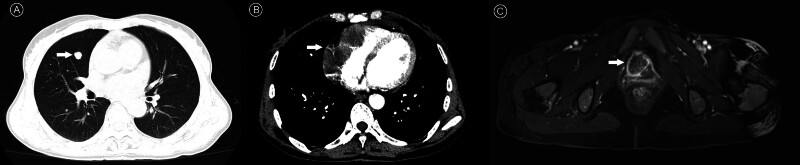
Baseline imaging evaluation of pulmonary, cardiac, and urethral involvement prior to treatment (May 22, 2024). (A) Chest CT shows a hyperdense pulmonary metastasis (arrow). (B) Cardiac CT reveals a hypodense cardiac metastasis (arrow). (C) Pelvic MRI (contrast-enhanced T1-weighted) demonstrates an enhancing urethral metastasis (arrow) with perilesional soft tissue enhancement. CT = computed tomography, MRI = magnetic resonance imaging.
